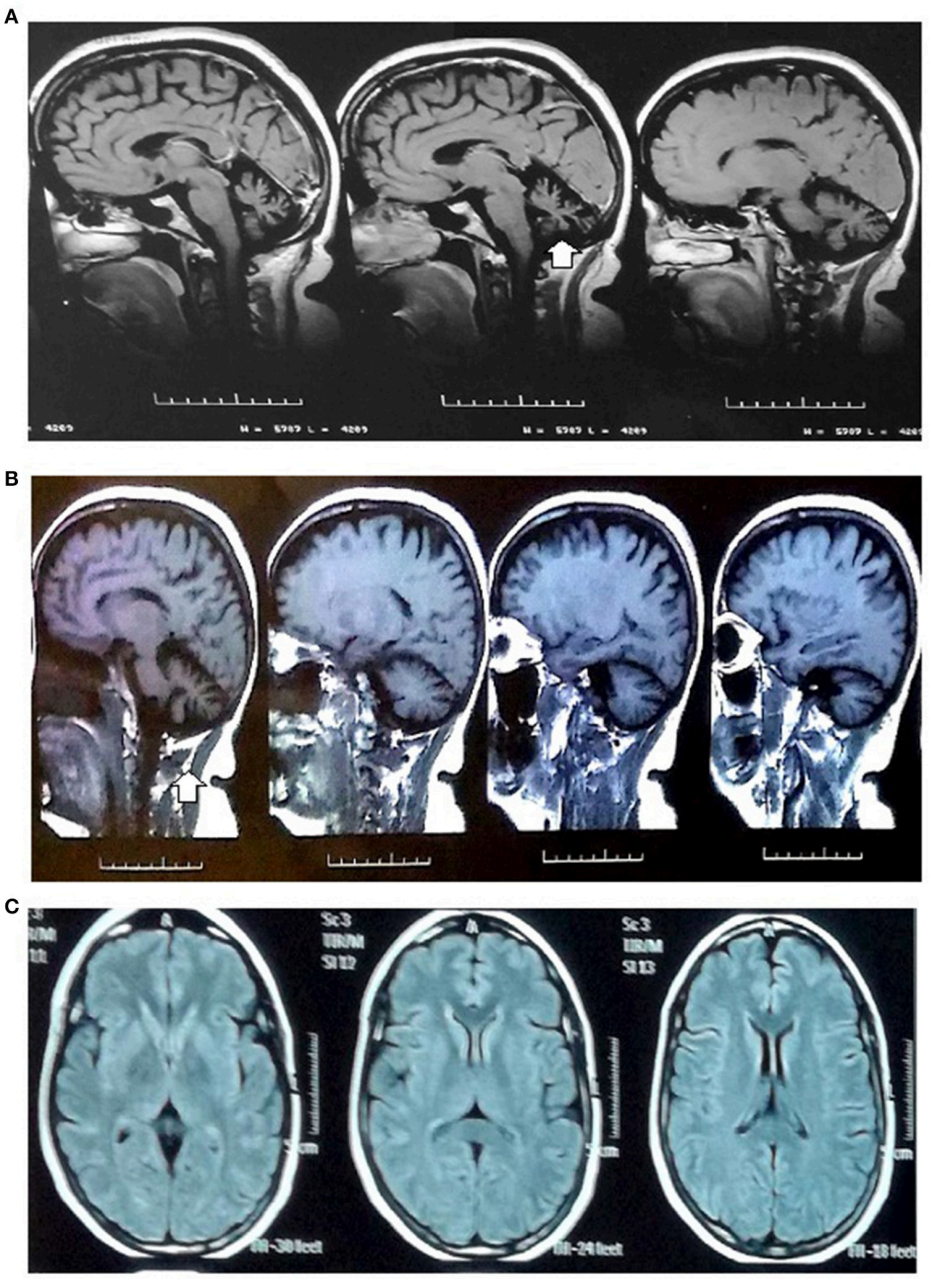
MRI images. MRI images (1.5 tesla): (A,B) Sagittal sections in the T1-weighted MRI images: global cerebellar atrophy with a marked enlargement of the subarachnoid space, thinning of cerebellar folds and vermis (arrow) related to diffuse cerebellar atrophy. (C) Axial section in the T1 sequence: cerebral white matter and ventricles without alterations.
